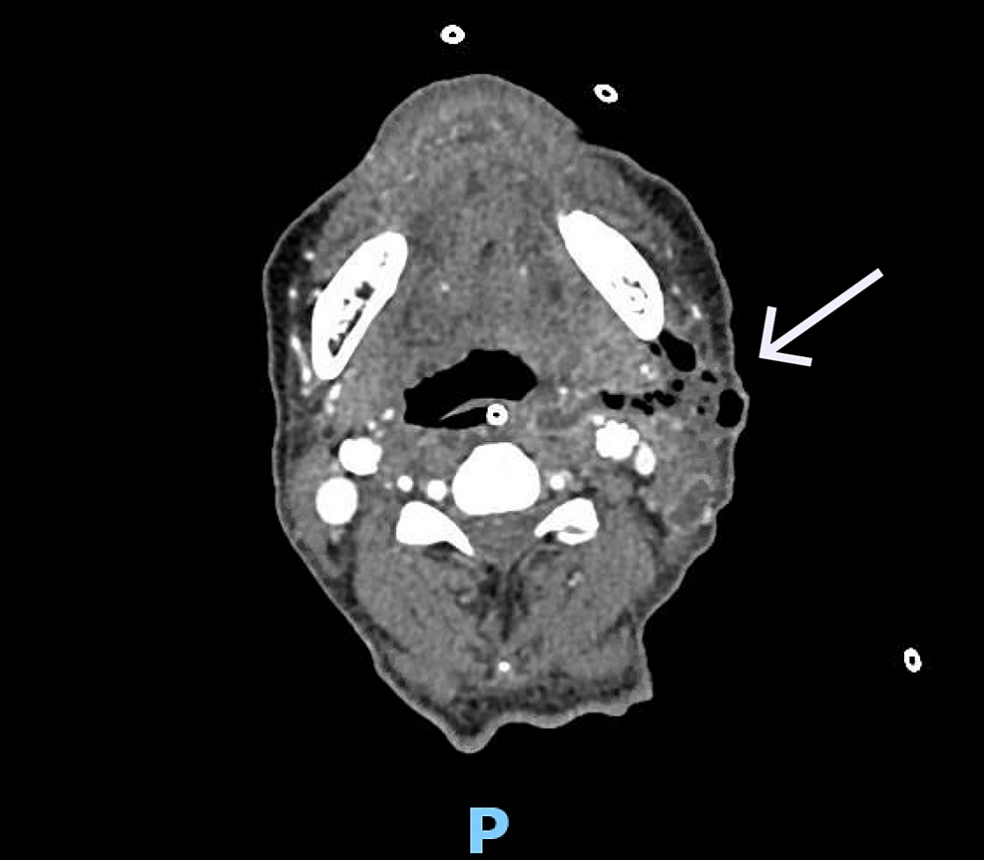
CT neck with contrast 20 days post-operatively showing a complex abscess in the left neck.
